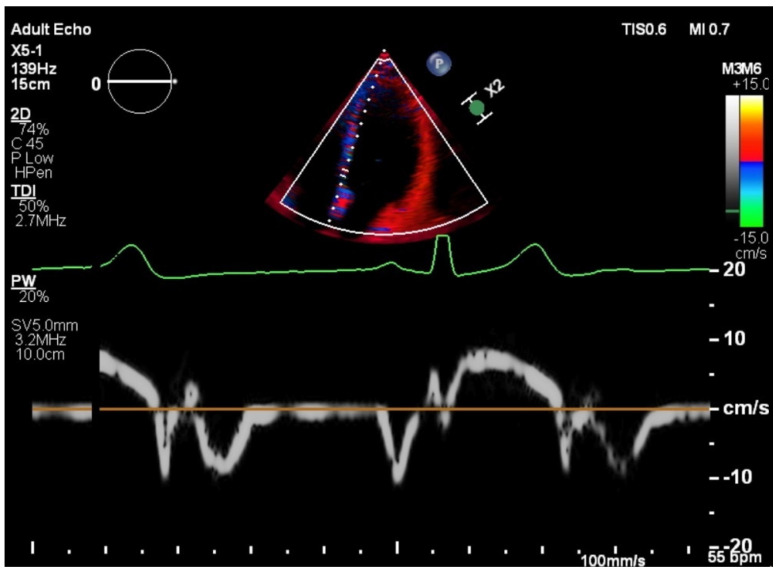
Tissue Doppler imaging echocardiography of a healthy pregnant patient in apical 4 chamber view, septal interogation–MV e’ Vel lat (mitral valve e wave velocity), MV a’ Vel lat (mitral valve a wave velocity), MV s’ Vel lat (mitral valve s wave velocity), E/E’ lat = relaxation ratio (E peak early mitral valve inflow velocity, E’ early mitral valve diastolic velocity). Courtesy of Elias Cardiology Department.
